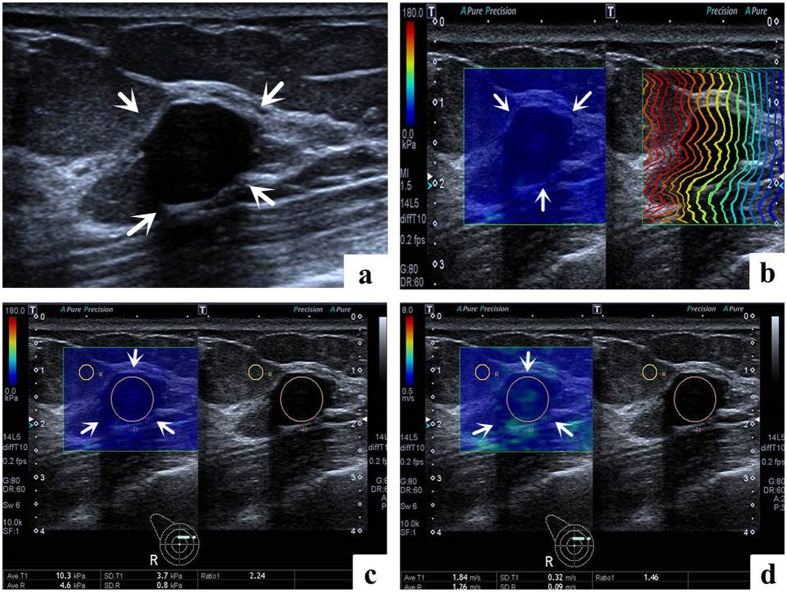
Images in a 50-year-old woman with fibroadenoma. (a) The lesion (arrows) is shown on B-mode ultrasound. (b) The lesion (arrows) shows regularly parallel contour lines on the shear wave propagation mode. (c) The Emean, E_SD_ and Eratio of the lesion (arrows) on elasticity mode are 10.3 kPa, 3.7 kPa and 2.24 respectively. (d) The Emean, E_SD_ and Eratio of the lesion (arrows) on shear wave speed mode are 1.84 m/s, 0.32 m/s and 1.46 respectively.
